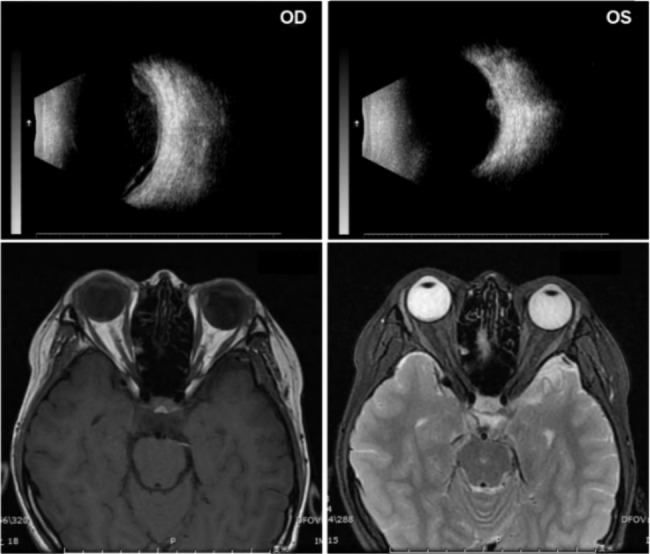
Ancillary Examination prior to treatment. (a): Ultrasonography; (b): Orbital Magnetic Resonance Imaging
